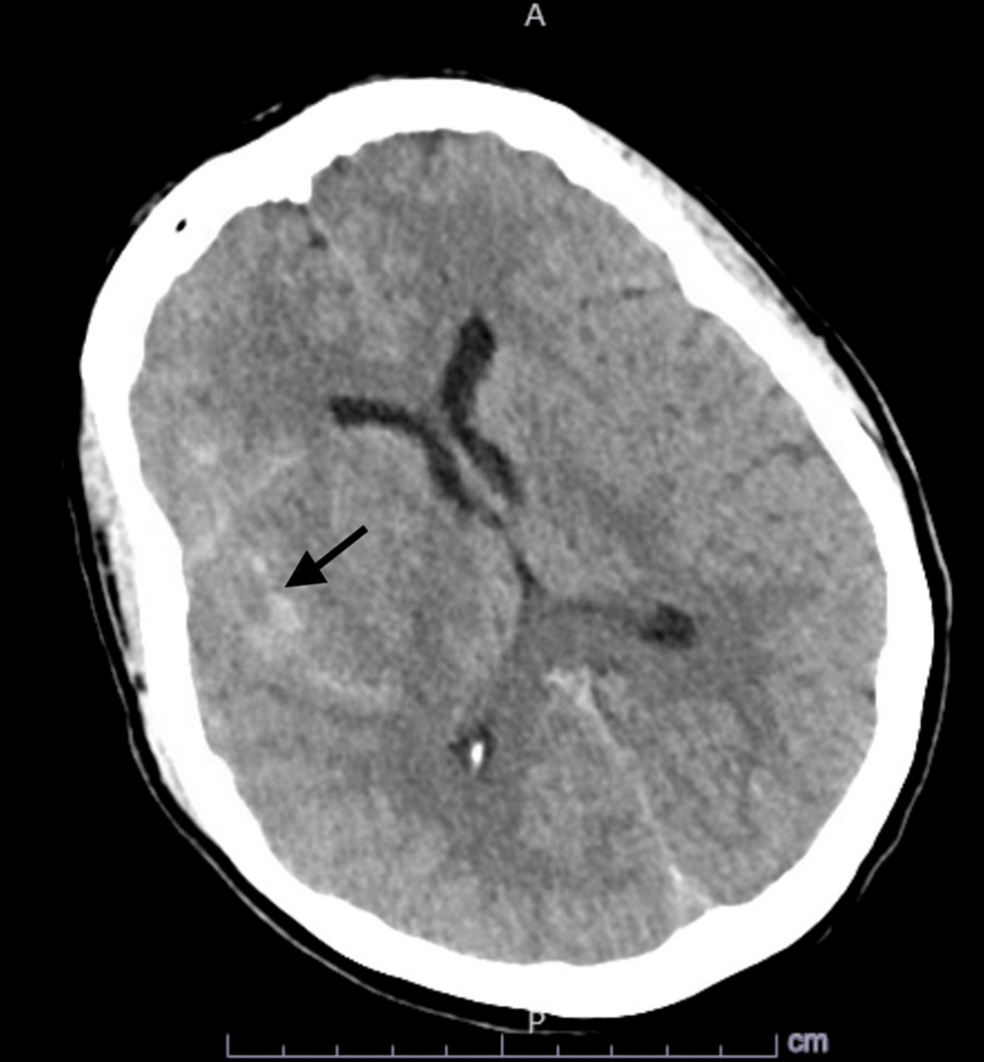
Parenchymal Extension of SAH Computed tomography (CT) head showing intraparenchymal extension of subarachnoid hemorrhage (SAH).
